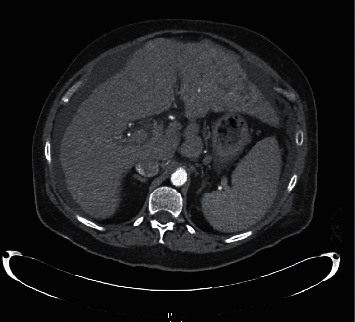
CT liver mass protocol with IV contrast showing evidence of cirrhosis with features of portal hypertension, an ill-defined infiltrative mass in left hepatic lobe suggestive of infiltrative HCC, hypoattenuating lesions in the right hepatic lobe, and periportal lymphadenopathy.
